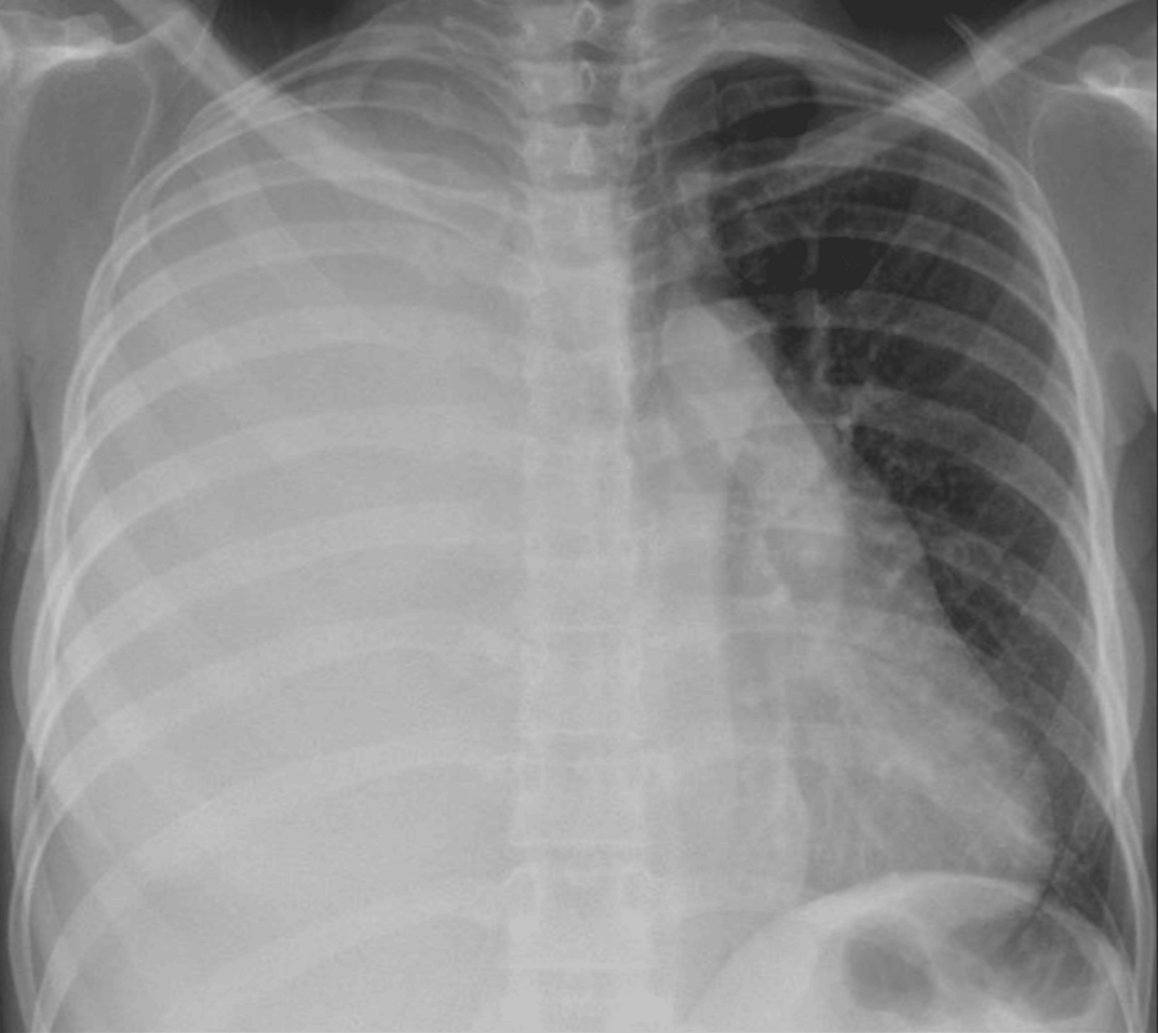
Chest X-ray on first presentation revealing a massive right-sided pleural effusion resulting in complete opacification of the right hemithorax and leftward mediastinal shift
